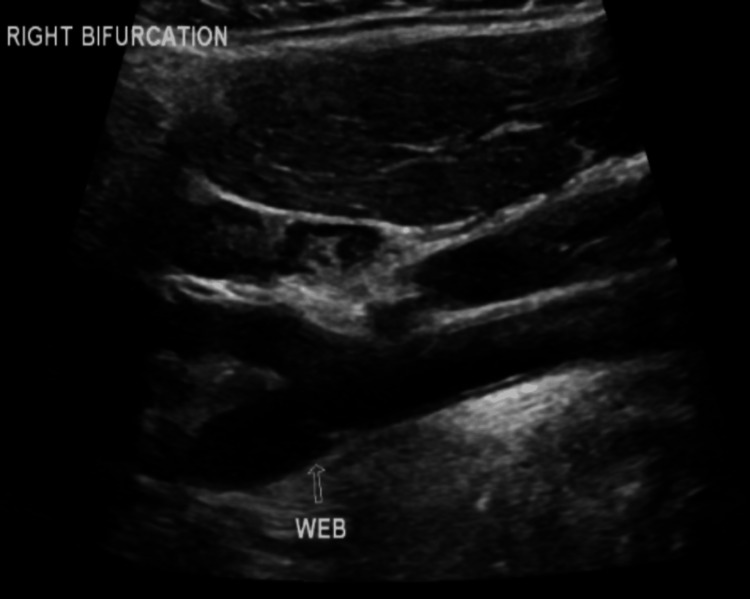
Proximal web only visible on B-mode of duplex ultrasound
